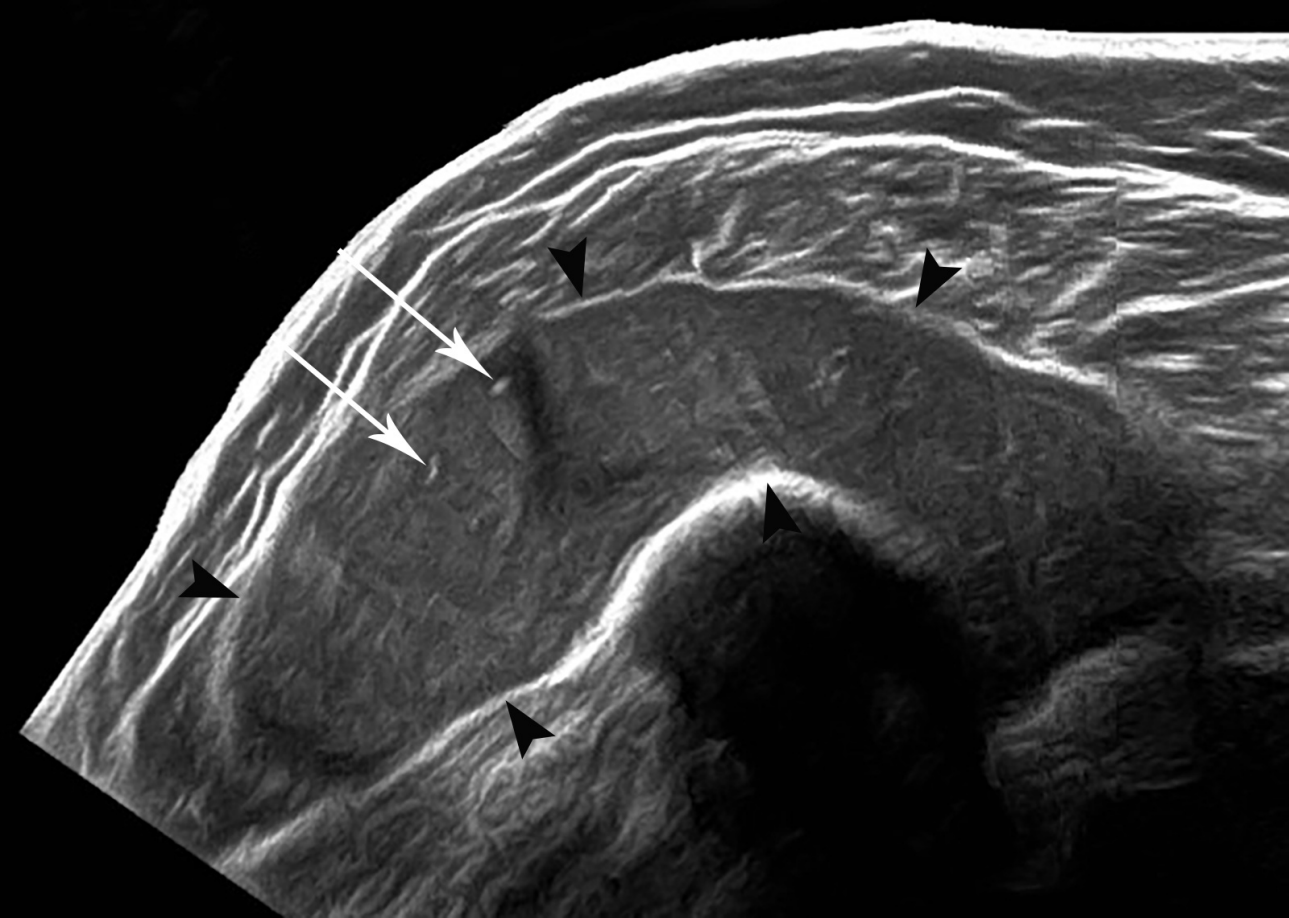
Grayscale Ultrasound (US) Imaging of the Right Shoulder in a 28-Year-Old Female with Shoulder Mass. US demonstrates a well-circumscribed, mildly hypoechoic 7.8 cm mass abutting the deltoid muscle (black arrowheads) with multiple internal hyperechoic 'flecks' (white arrows).
